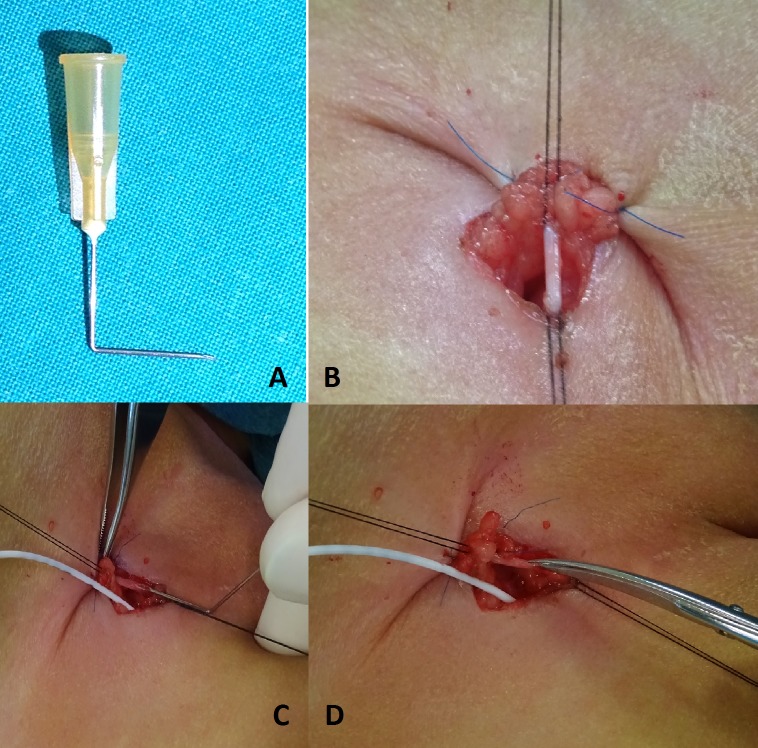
Figure 1: (A) CT scan showing huge cyst. (B) Intraoperative finding.
